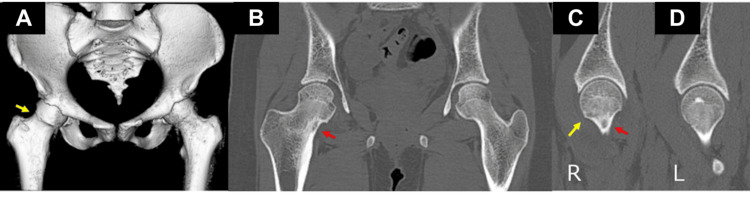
Preoperative computed tomography (CT) images The yellow arrow shows the bony bump on three-dimensional CT (A) and sagittal CT images (C). There was no bony bump on sagittal CT images (D). The red arrows show a nidus (B, C).
